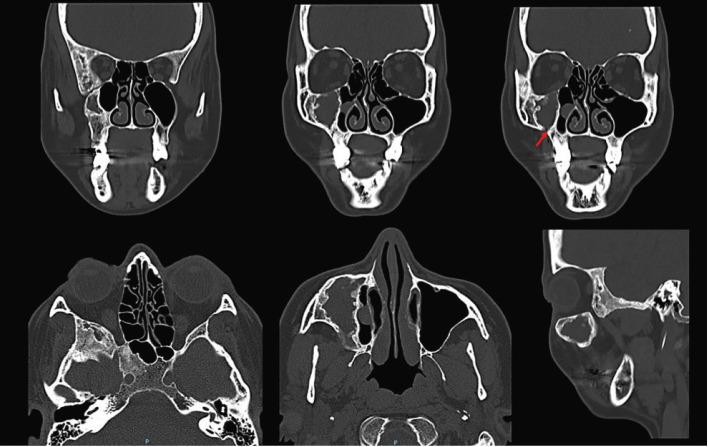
CT performed after the surgical procedure, there was no relevant alterations in the radiographic features when compared to the previous exams (Fig. 1), except for an area which corresponds to the surgical access (red arrow).
